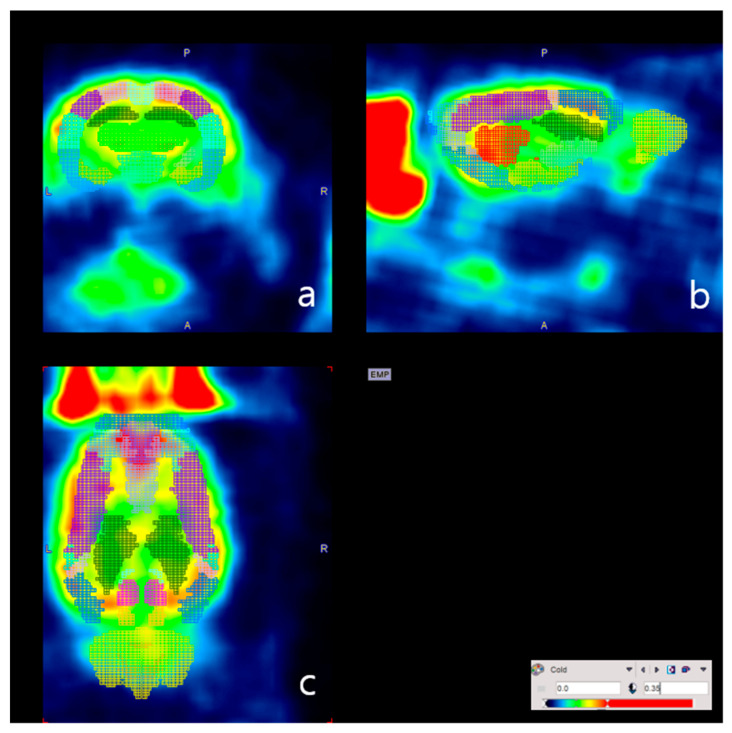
Quantification of FDG brain uptake of each cerebral region. Representative images-co-registered PET atlas images for one subject-are shown in the (a) coronal, (b) sagittal, and (c) axial orientations.
